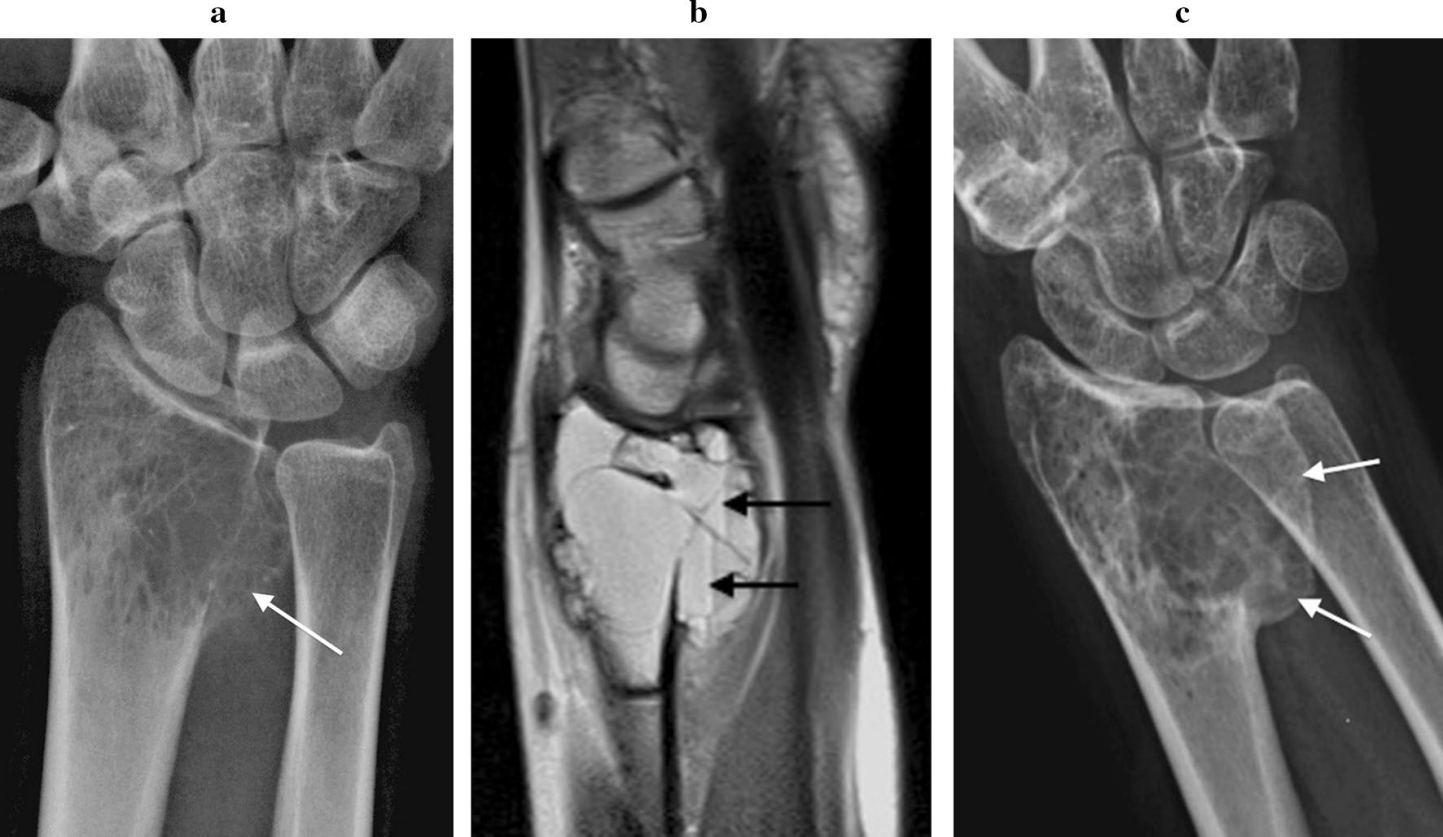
Patient 2: pre denosumab, a frontal plain radiograph shows a lytic Campanacci grade 2 distal radial GCTB with medial expansion and cortical thinning (arrow). b Sagittal T2-weighted MR image demonstrates volar expansion and fluid fluid levels (arrows) indicating secondary aneurysmal bone cyst. Post denosumab at 2 months, c frontal plain radiograph demonstrates more clearly defined sclerotic tumour margins particularly along the expansile medial aspect of the lesion (arrows) and mild matrix osteosclerosis
